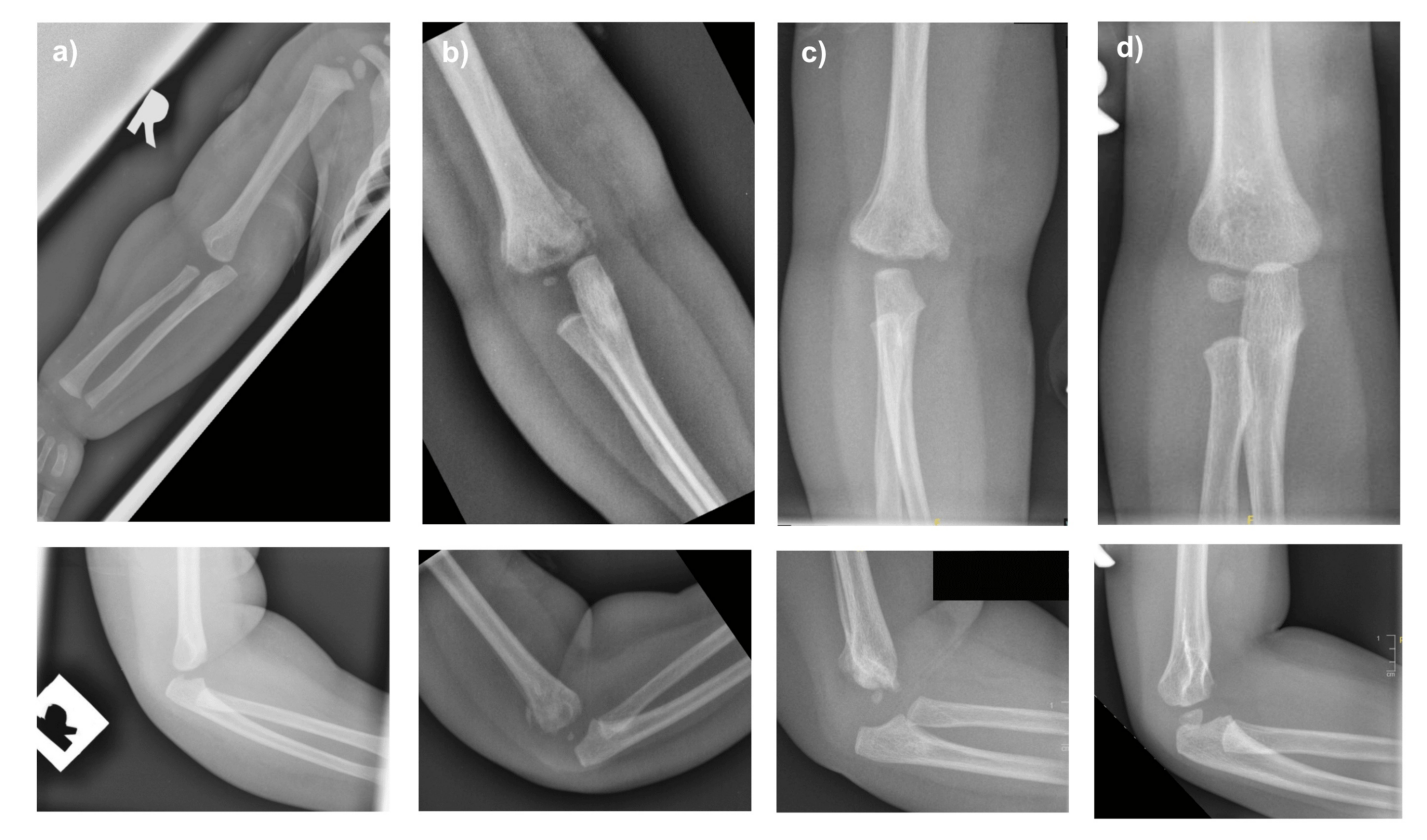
Serial elbow radiographs of case 1 Serial elbow radiographs at: (a) initial injury: showing posteromedial displacement of radius and ulna relative to distal humerus; (b) 3 weeks: showing callus formation and posterior metaphyseal spike; (c) 6 weeks: showing united fracture with residual posterior displacement of the forearm; (d) 18 months: showing a remodelled distal humerus.
